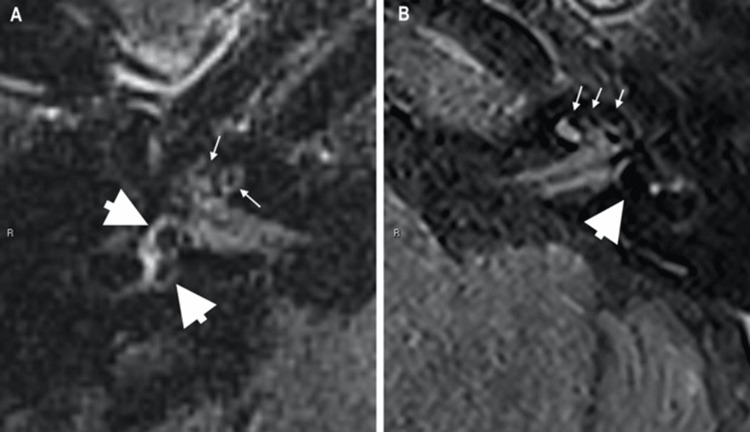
Radiographic evaluation of membranous labyrinth distension. A: axial view of the right ear MRI, classified as grade I. B: axial view of the left ear MRI, classified as grade III. Smaller arrow: cochlear distension; larger arrow: vestibular distension.
